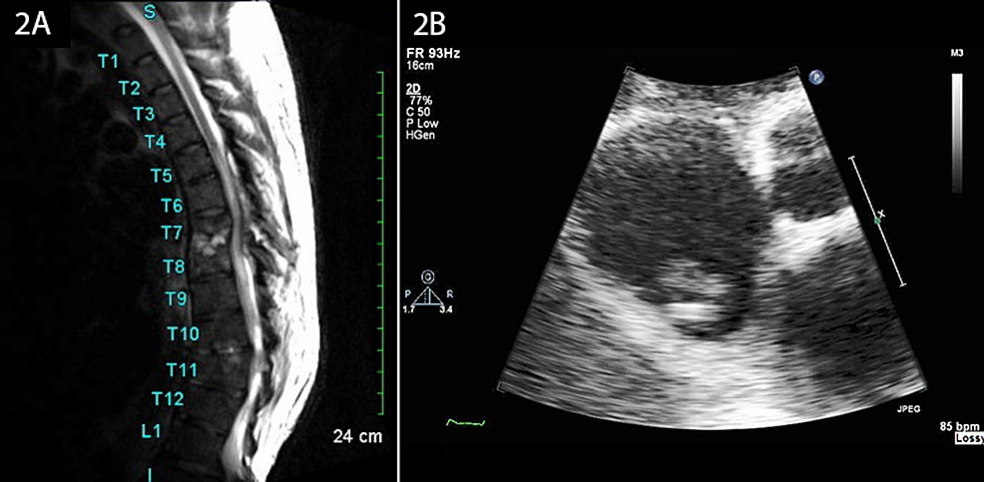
MRI of the thoracic spine and transthoracic echocardiogram. (A) Sagittal MRI of the thoracic spine confirming osteomyelitis with disc space infection at T7-T8 and similar, although much earlier, process at T10-T11. (B) An apical view of a two-dimensional transthoracic echocardiogram reveals vegetation on a eustachian valve remnant in the patient’s right atrium. MRI = magnetic resonance imaging
